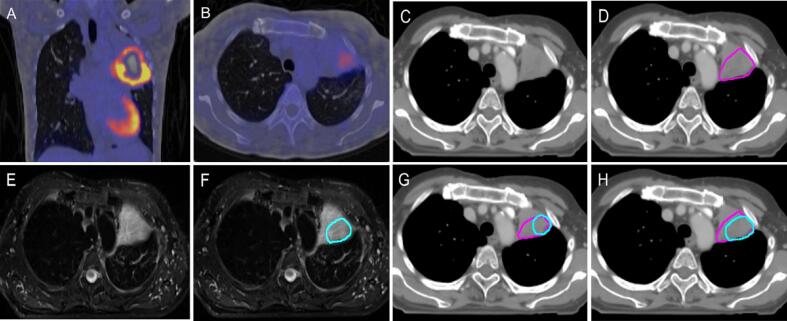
Atelectasis and lung collapse; (A) Coronal image of PET-CT scan showing left apical collapse, (B – F): Same transverse slice at the superior end of the tumour on (B) PET-CT, (C) CT scan (L: 46, W:600 HU) with no CT median contour (D) CT scan with CT median contour (pink), (E) T_2_w TSE fat-sat image without MR median contour, (F) T_2_w TSE fat-sat image with smaller MR median contour (light blue). T_2_w TSE fat-sat image demonstrates more tumour heterogeneity (E) compared to CT (C), although the panel agreed that the edge of the GTV was still challenging to define. (G – H): The two consecutive most apical slices of the tumour on CT scan (L: 46, W:600 HU), (G) is the most superior slice, followed by one consecutive slice (H) moving caudally, showing CT consensus contour (pink) and smaller MR consensus contour (light blue) with more generous CT consensus contour compared to MR consensus contour. (For interpretation of the references to colour in this figure legend, the reader is referred to the web version of this article.)
